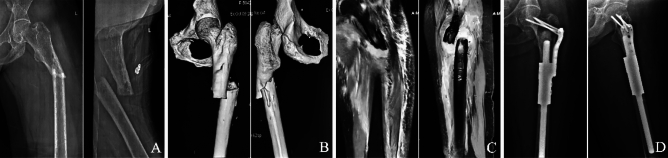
A female aged 51 years, left proximal femur metastasis from unknown origin. (A) Anteroposterior and lateral radiographs of a left femur showing a pathological diaphyseal fracture due to metastatic tumor. (B) 3D reconstruction by CT showing a pathological diaphyseal fracture, and fracture end was irregular. (C) Fat-suppressed T2-weighted (T2W) images showing a pathological diaphyseal fracture which had intramedullary hematoma with fat necrosis and peripheral hematoma. (D) Anteroposterior and lateral radiographs showing that modular intercalary prosthetic reconstruction after tumour resection.
